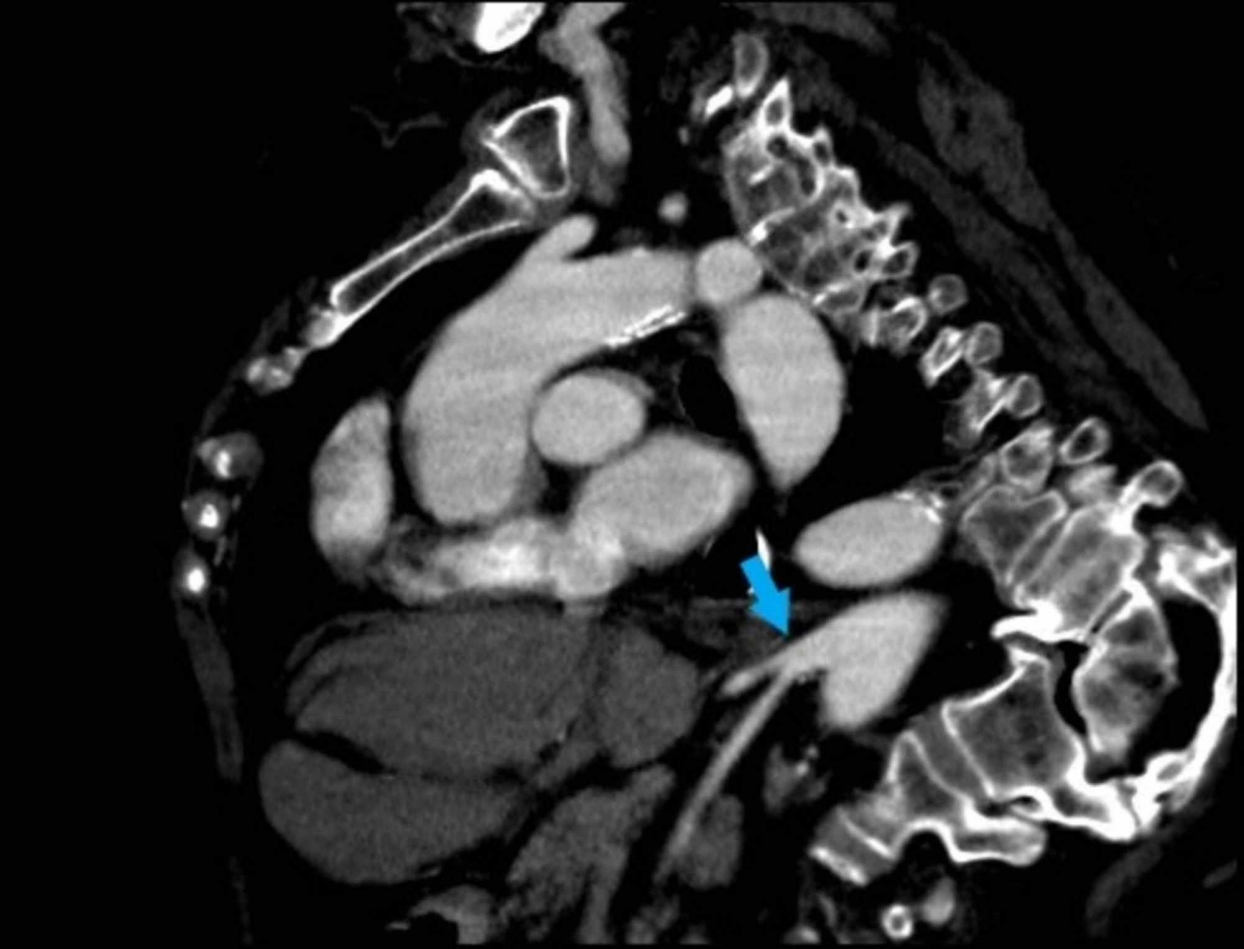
Chest and upper abdomen contrast-enchanced computed tomography (CT) in the venous phase, sagittal view, showing a common celiacomesenteric trunk (arrow).
